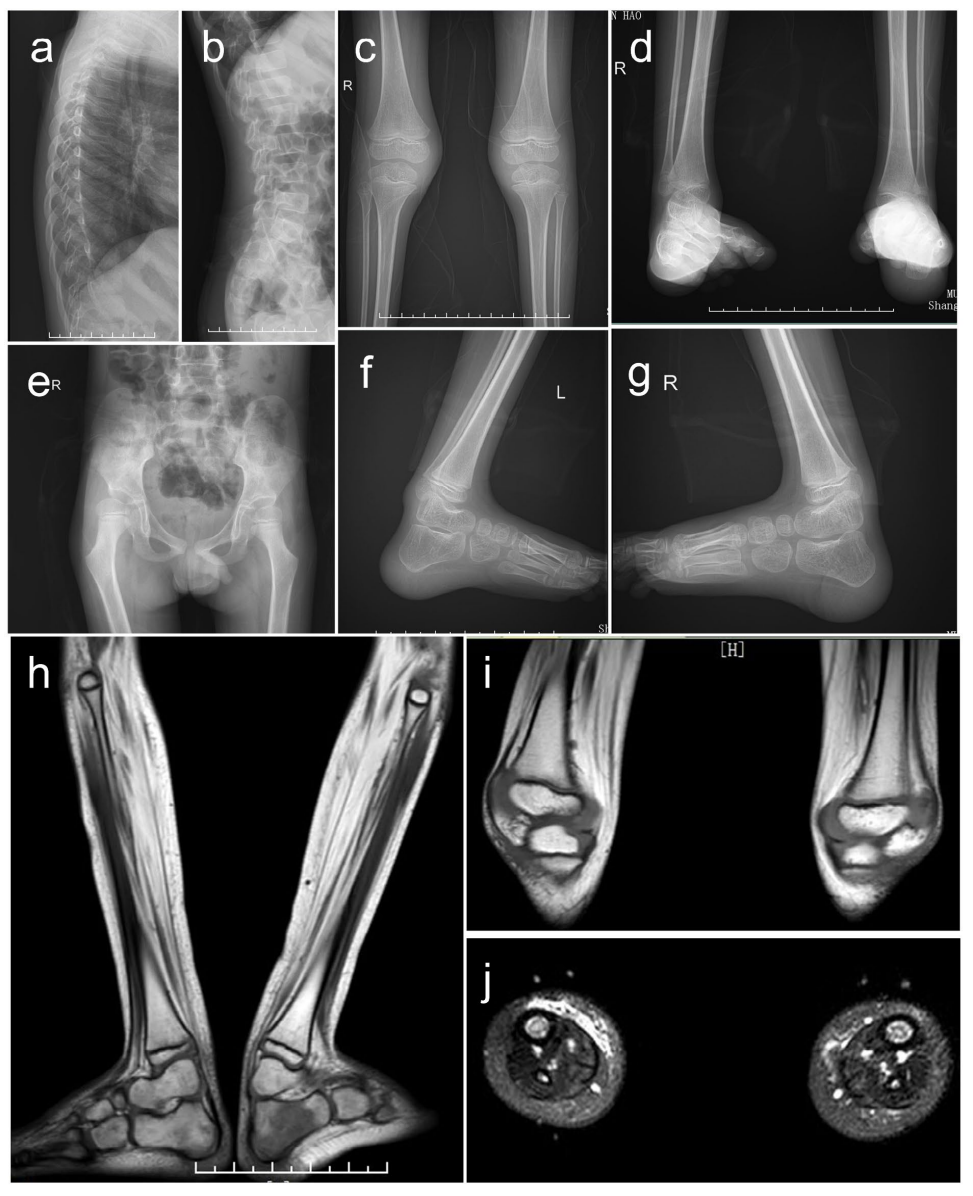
X-ray radiography and leg muscle MRI of the proband. (a, b, e) X-ray radiography of thoracic and lumbar vertebrae as well as hips was normal and showed no fractures. (c, d, f, g) X-ray radiography of the knees showed soft tissue swelling and ankles showed talipes calcaneovarus and poor alignment of the tibial talus joints. (h) Coronal T1W MRI of calf muscle with severe atrophy and fatty infiltration. Bone marrow oedema of the left calcaneus resulting from the ankle fixation braces that the proband had worn for a long time can be seen. (i) Coronal T1W MRI of distal thigh muscle with severe atrophy and fatty infiltration in posterior thigh muscles. (j) Axial T2W MRI of calves with severe atrophy and subcutaneous fat thickening
